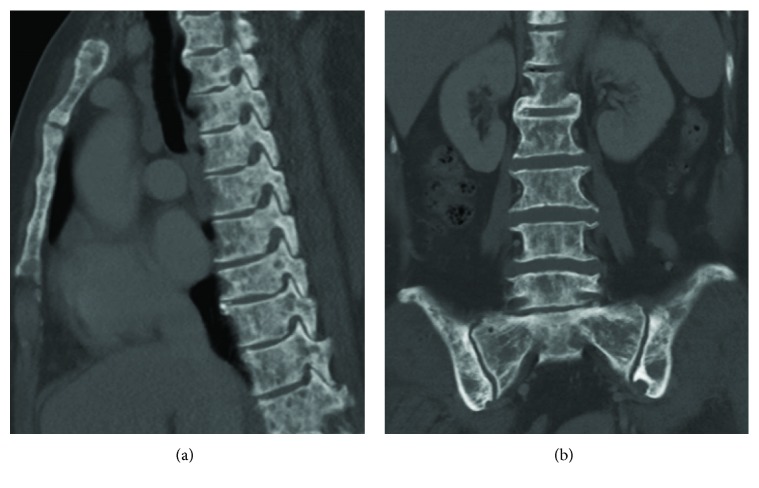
Chest and abdomen CT images on bone window, September 14, 2017. Multiple osteosclerotic and osteolytic lesions involved the sternum, vertebra, sacrum, and bilateral iliac bones.
